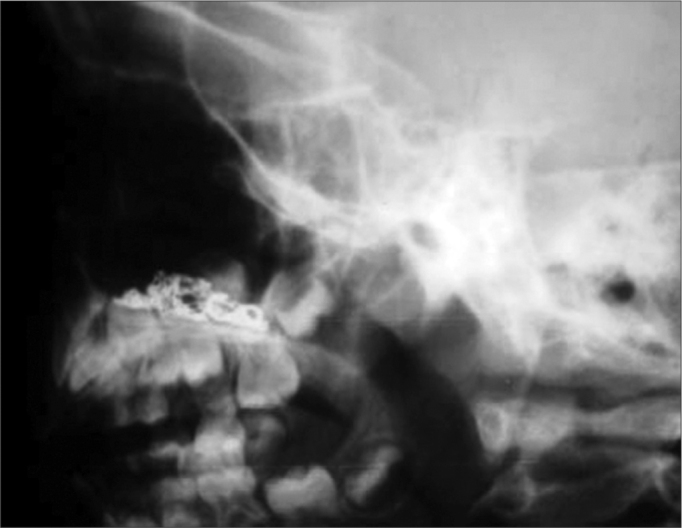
Simple fronto-nasal and side view x-rays.•Nasal endoscopy, with the 2.7 rigid endoscope (ideal for children) or 4mm and 0 or 30° angle, or flexible nasopharyngoscopy. This is the best method for both the diagnosis and removal of foreign bodies in dubious cases. In some cases general anesthesia is required^6^, ^7^. Most nasal foreign bodies are located in the anterior portion of the nasal cavities, due to inferior turbinate extension (Figure 2)^6^. Such fact makes most nasal cavity foreign bodies of relative easy removal, in experienced hands. The ideal position for removal is having the patient sitting, on the lap of the person responsible, who will hold the child's arms and legs. A helper holds the head, which should be mildly extended (about 30°) (Figure 3). In some cases of difficult containment or a technically more challenging removal, general anesthesia may be necessary. In our experience we prefer the following instruments:
